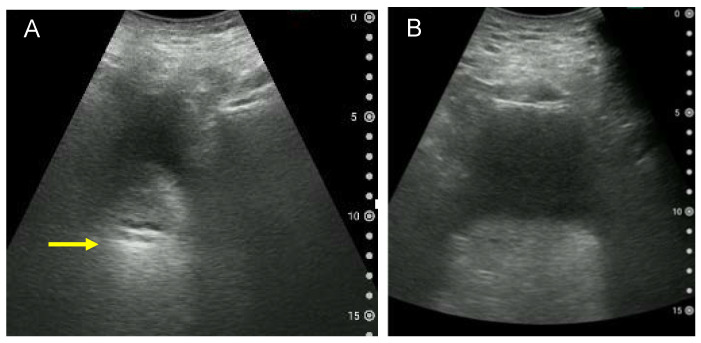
Examples of a blurred ultrasound image of the bladder and rectum. (A) Blurred transverse ultrasound image of the rectum due to little urine in the bladder and intestinal gas. A hyperechoic area is observed, possibly indicating faecal retention (arrow). (B) Transverse ultrasound image of the rectum in an adult male with a body mass index of 28.6 kg/m^2^. Due to the thickness of the abdominal wall, the bladder and rectum cannot be clearly observed. The volume of urine voided immediately after imaging is approximately 180 mL.
